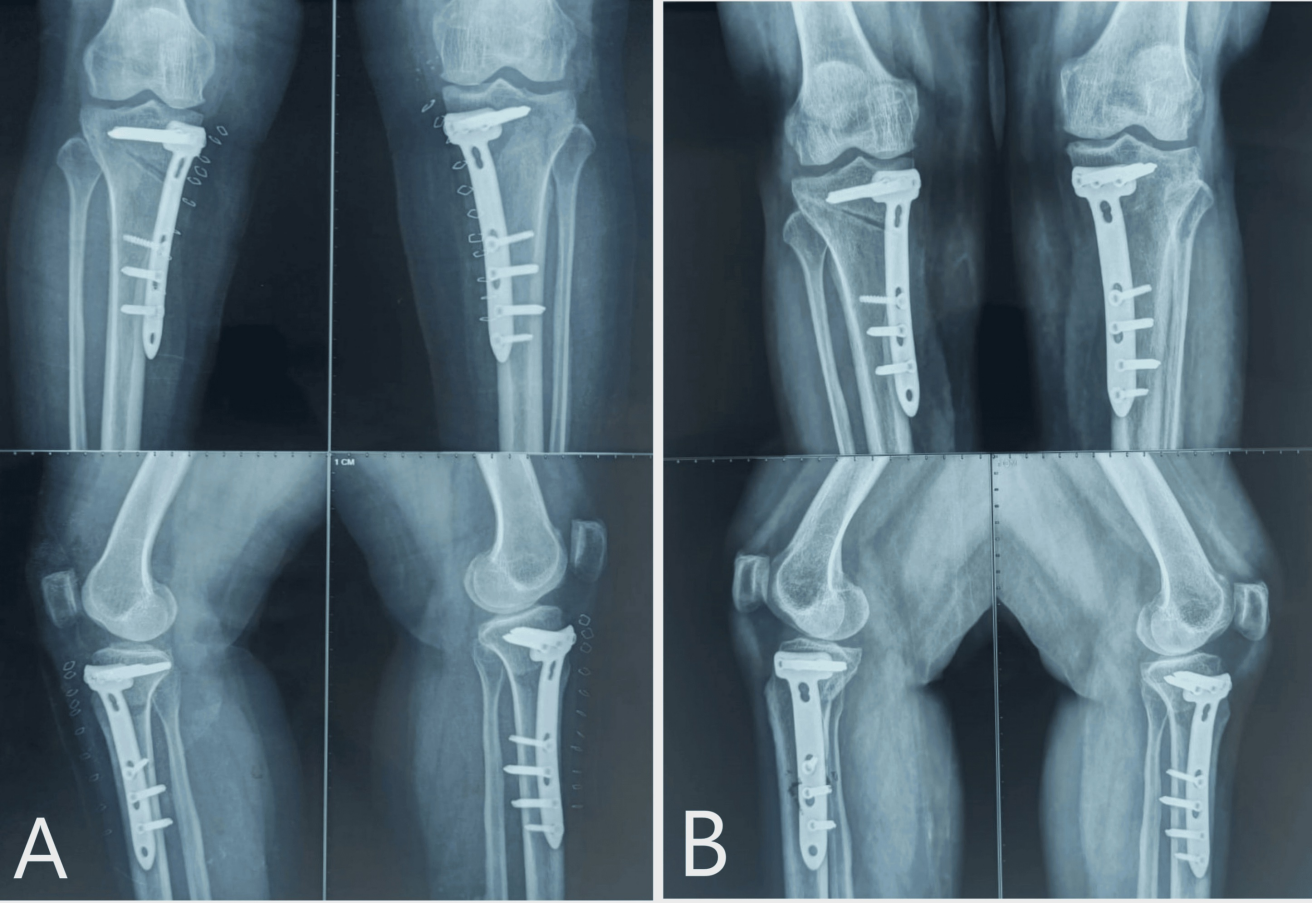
A) Immediate post-operative radiograph of MOWHTO using Dr Saigal's plate. B) Six months of follow-up X-ray showing progress of union in both knees.
